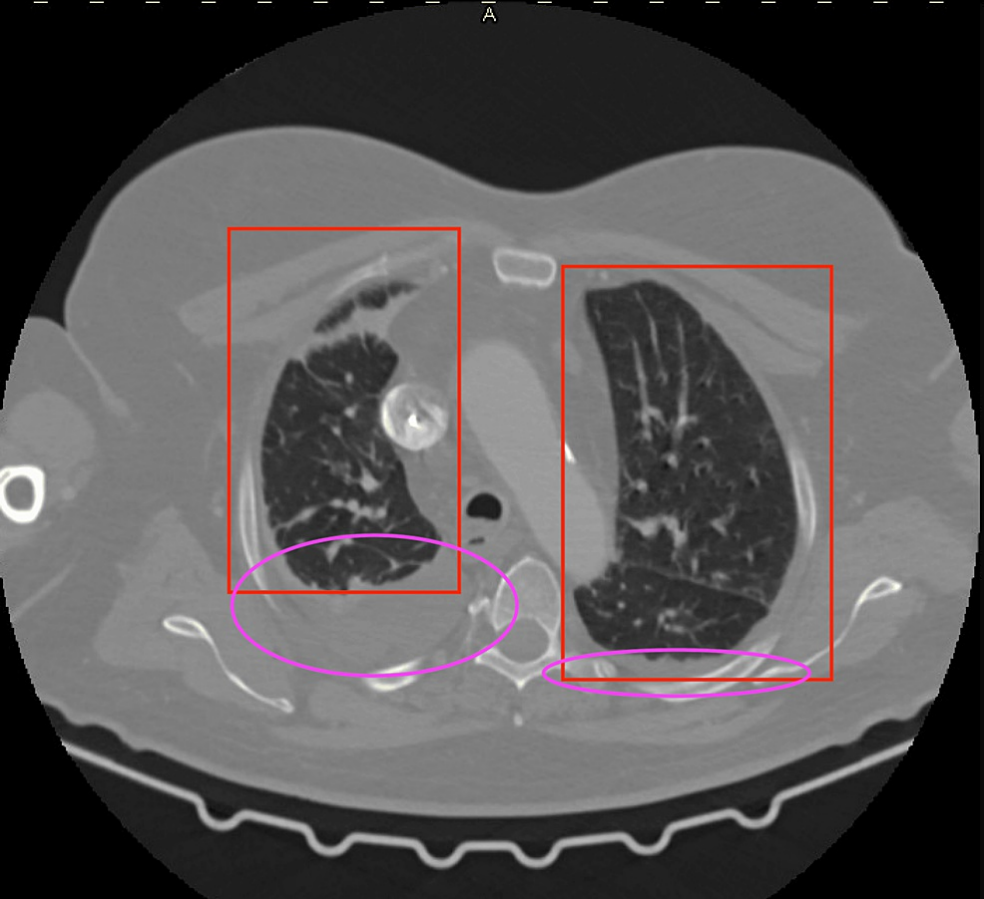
CT scan of the lungs. Upper pulmonary lobes Pink circles: presence of fluid; Red rectangles: typical inflammatory image of lungs after COVID-19 infection
